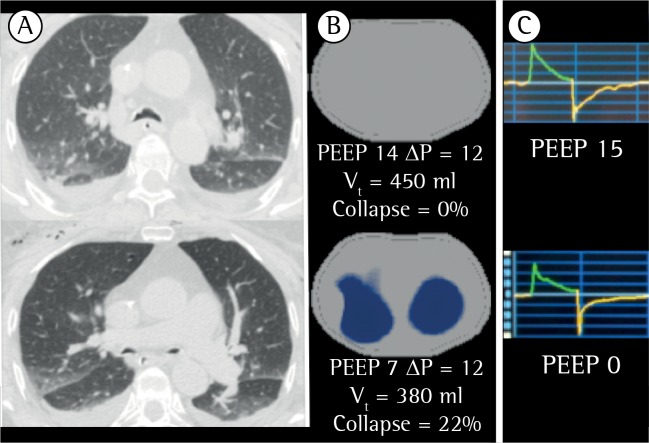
In A, an HRCT scans of the chest showing bilateral small pleural effusions with partial lower lobe atelectasis, and a reduction in the cross-sectional diameters of the trachea and main bronchi, mainly in the subsegmental divisions of the lower lobes, bilaterally; in B, electrical impedance tomography scans showing that the use of a higher PEEP prevented airway collapse an expiratory flow curve indicative of airway obstruction; in C, an expiratory flow curve indicative of airway obstruction and a dramatically improved expiratory flow curve, no longer indicating flow limitation, after an increase in the positive end-expiratory pressure (PEEP).
